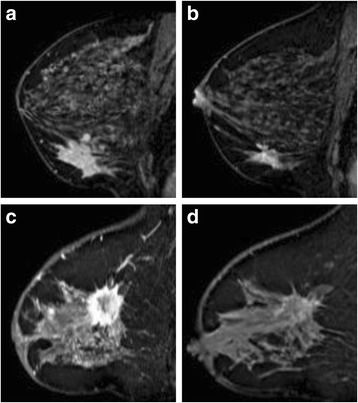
Example of a tumor that shrinks concentrically: magnetic resonance imaging (MRI) before neoadjuvant chemotherapy and immunotherapy (NAC) (a) and halfway through NAC (b); and a tumor that crumbles: MRI before NAC (c) and halfway through NAC (d)
